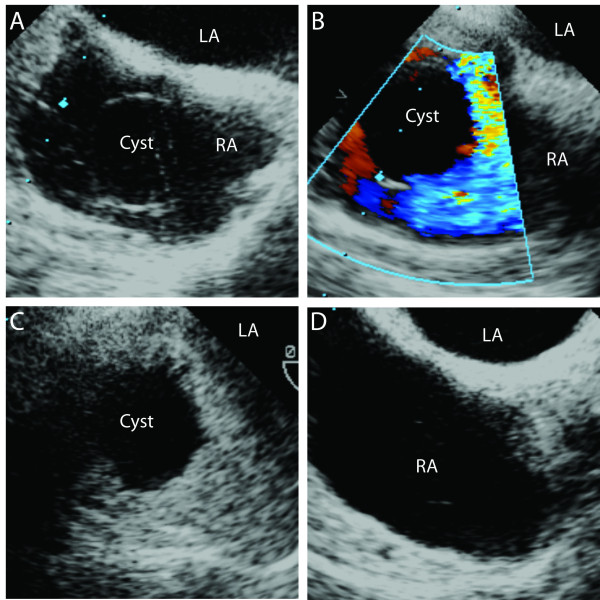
In a modified midesophageal bicaval view, a cyst was seen in the right atrium. Panel A: A cystic structure in the RA with a thin wall resulting in difficult visualization. Panel B: Color Doppler was used to confirm the existence of the cyst close to the IVC. Panel C: Bubble study, used to exclude PFO, confirmed the borders of the cyst and its position close to the IVC intersection. Panel D: A completely empty RA after the removal of the tumor. RA: right atrium, LA: left atrium, IVC: inferior vena cava.
